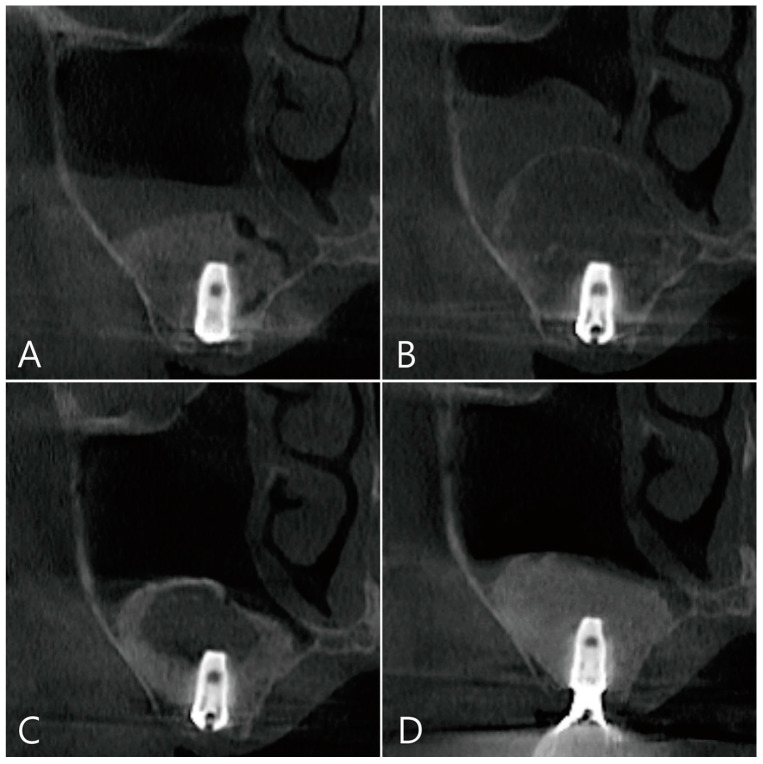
Coronal images of the CBCT were taken at multiple points during the healing process after surgery: (A) an image taken immediately after surgery; (B) an image taken one week after surgery. A large grafting void occurred over the apical half of the implant, and thickening of the maxillary sinus mucosa was also observed; (C) in the image 6 months after surgery, the size of the grafting void was slightly reduced. The void presents with a cystic appearance resembling SCC. The apical bony support of the implant was lost. The grafting void was removed and additional bone grafting was performed; (D) in the CBCT image taken 6 months after the prosthesis was delivered, it can be confirmed that the previous grafting void was replaced with a bone graft substitute.
